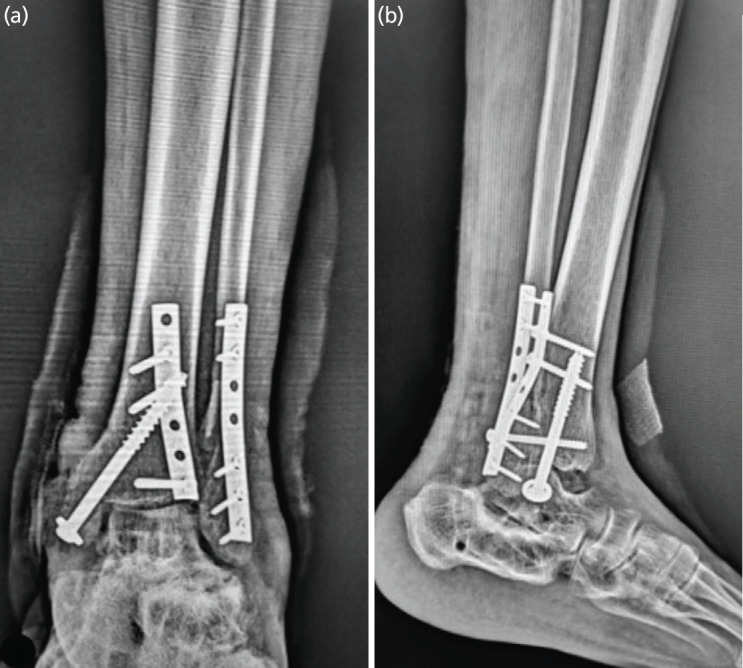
Post-operative radiographs of the same patient; (a) shows the anteroposterior view showing the posterior malleolus fracture fragment fixed with a semitubular plate in buttress mode; (b) shows the lateral view of the same.
